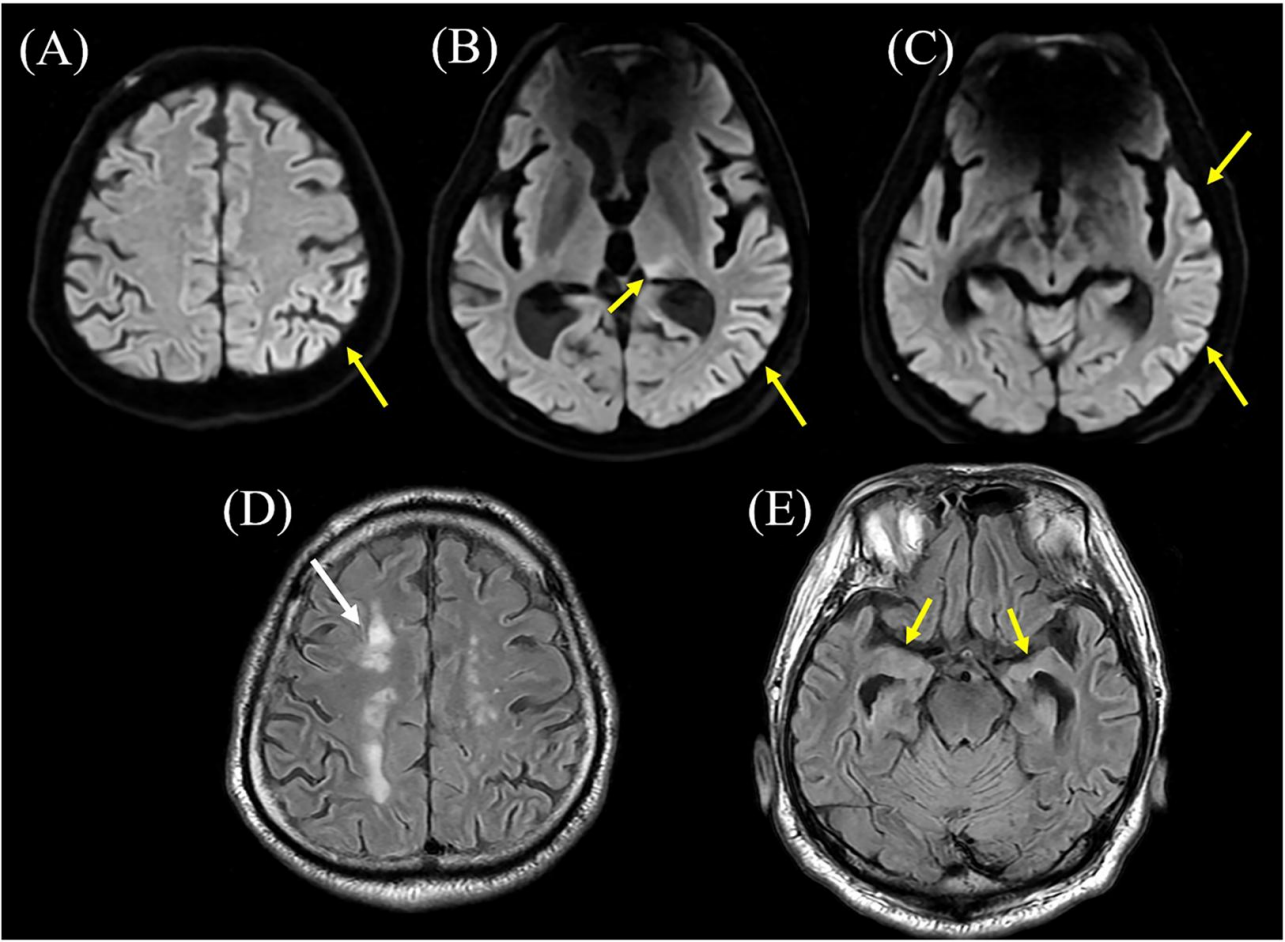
Brain magnetic resonance imaging (MRI) result. (A–C) Diffusion-weighted MRI shows hyperintensity in the left temporal and parietal lobes involving the thalamic pulvinar (arrows). These findings were resolved on follow-up imaging on day 16 (data not shown). (D,E) T2-FLAIR MRI reveals deep white matter lesions in the watershed region (white arrow) and atrophy of the medial temporal lobes, particularly the hippocampus (yellow arrows).
